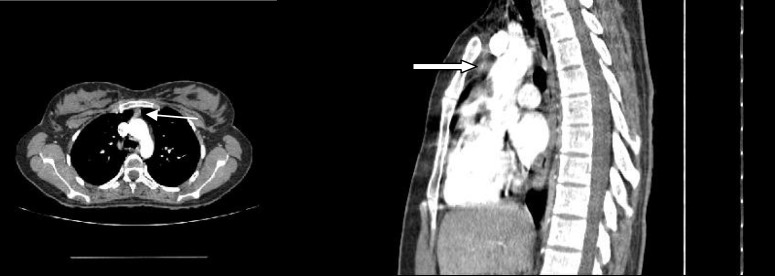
Axial CT scan with sagittal reconstruction from mediastinum in a 24 years old lady. A round centrally enhancing mass lesion in anterior mediastinum measuring 7.7 mm in transverse and 6 mm in anteroposterior diameter (white arrows). The images are taken with BrightSpeed GE 16 slice CT scanner, TI: 791 ms, kV: 120, mAs: 4, WL: 40, WW: 400, Slice thickness: 1.25mm
